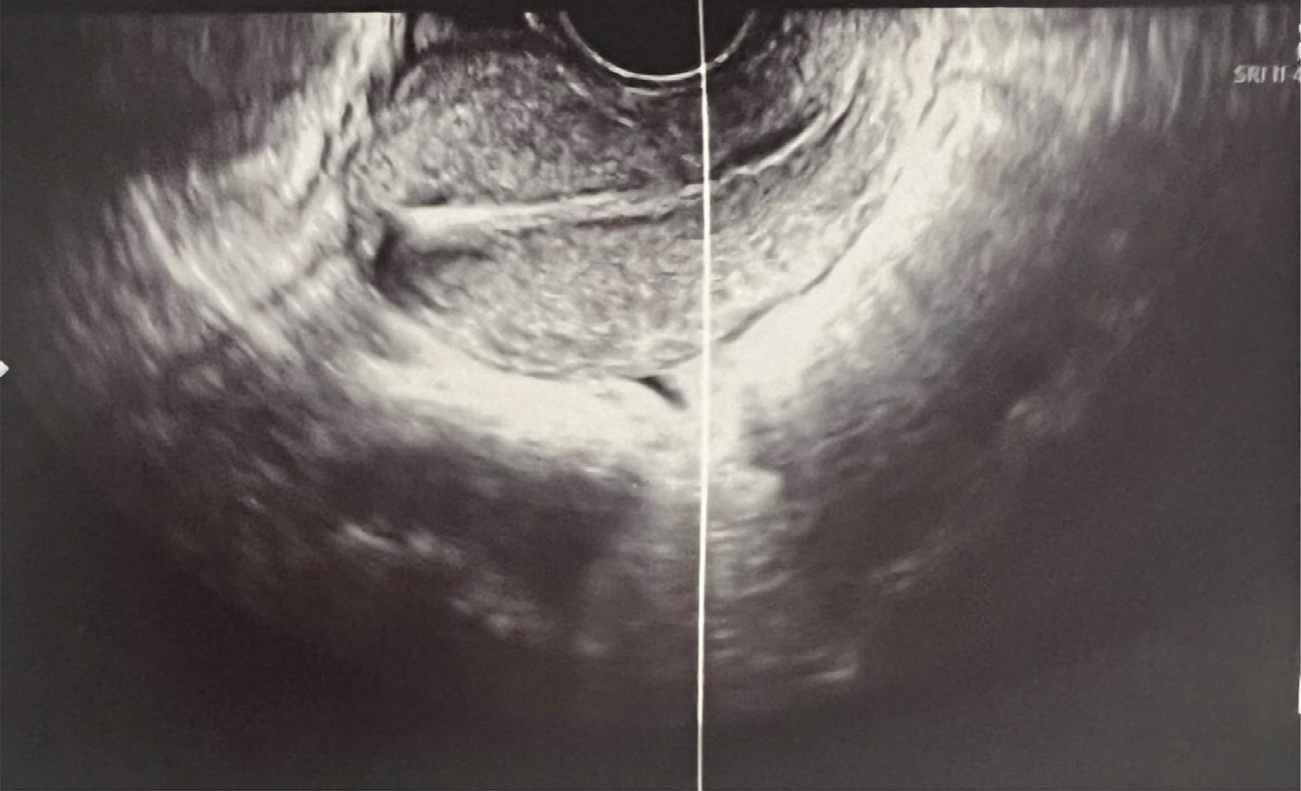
A pelvic ultrasound revealed a normal-sized uterus measuring 66*37 mm with homogeneous echostructure and regular contour. An intrauterine device (IUD) was observed intra uterine, positioned 17 mm from the uterine fundus.
